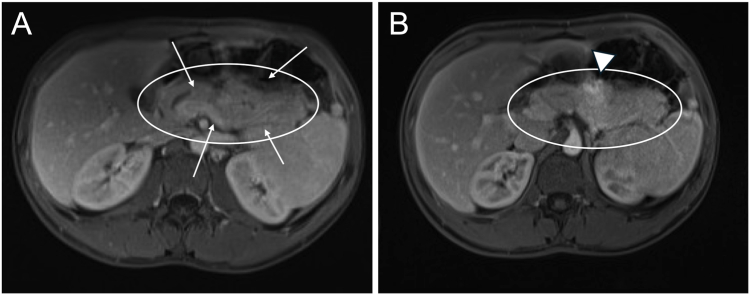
Magnetic resonance imaging of the abdomen obtained (A) before and (B) after steroid therapy reveals interval atrophy of the gland with resolution of the peripancreatic hypodense rim. Circles outline the pancreas. Arrows point to diffuse parenchymal swelling (sausage-shaped pancreas), which persists. Arrowhead points to a focal inflammatory mass.
